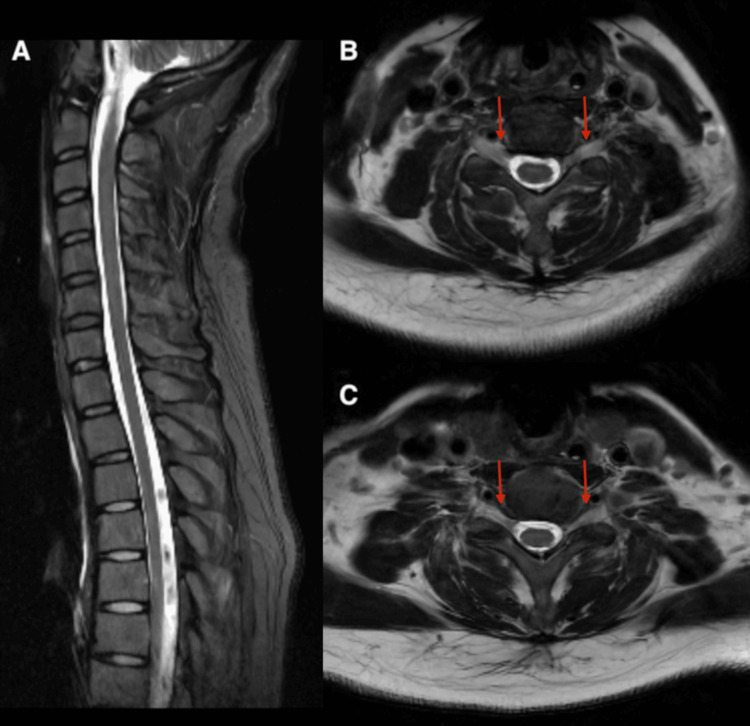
T2-weighted image of cervicothoracic spine MRI: (A) sagittal view, (B) axial view at the C5/6 level, and (C) axial view at the C6/7 level No structural abnormalities were observed on the sagittal view. (B) and (C) show bilateral enlargement of the nerve roots (red arrows).
